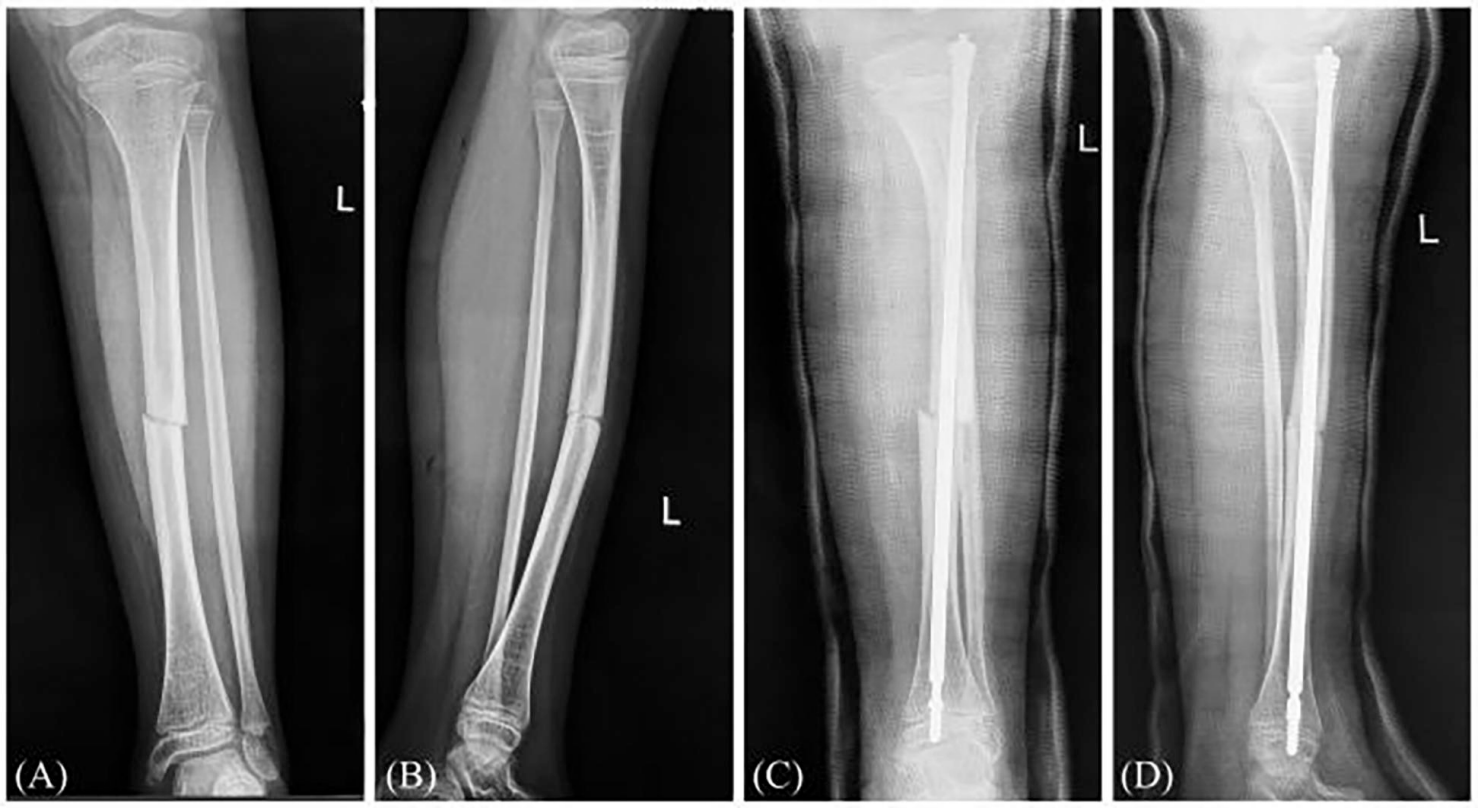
Radiographs of the left tibia in a 9-year-old patient. (A,B) Anteroposterior and lateral views showing a diaphyseal fracture of the left tibia. (C,D) Immediate postoperative anteroposterior and lateral views following FD nail internal fixation of the left tibia.
